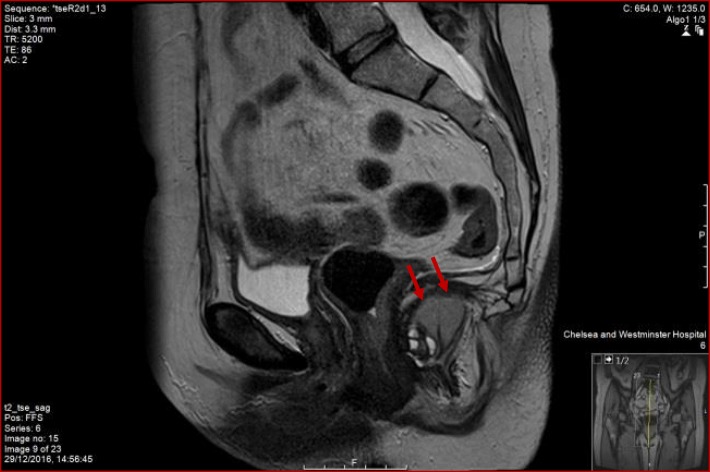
MRI scan image—sagittal view
